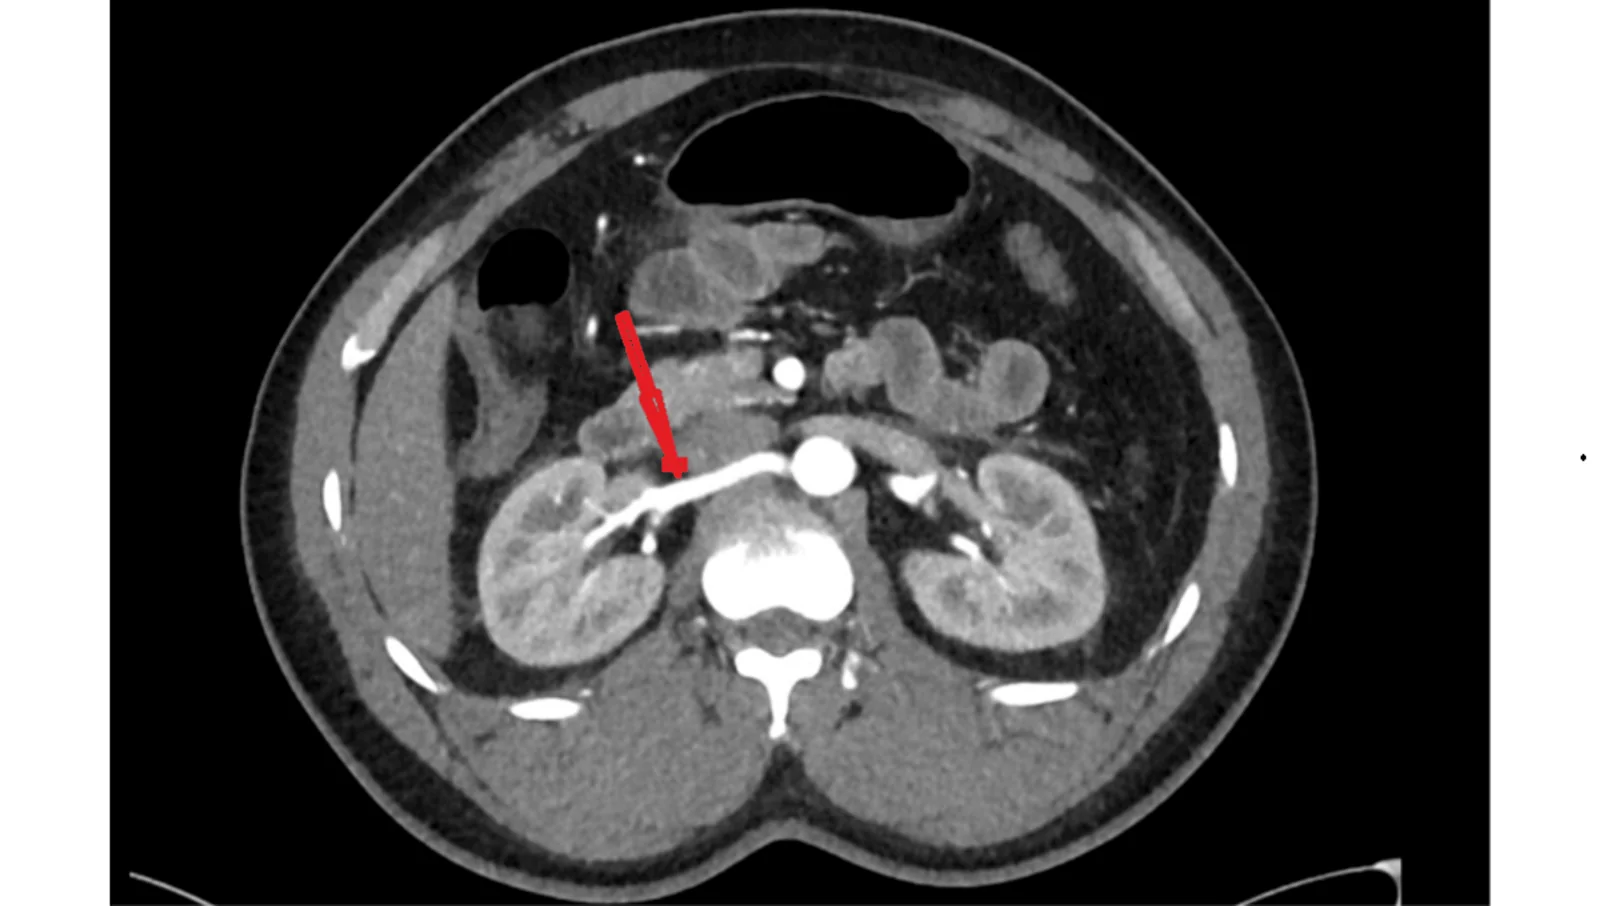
Subsequent CT angiography showing patent renal vasculature (arrows)
